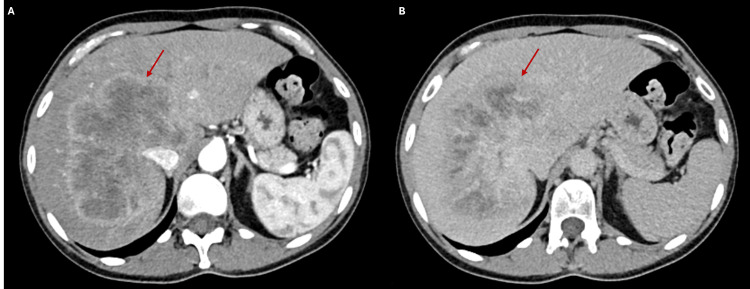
Initial CT showing a large liver lesion in the right lobe: (A) arterial phase and (B) delayed phase CT: computed tomography
